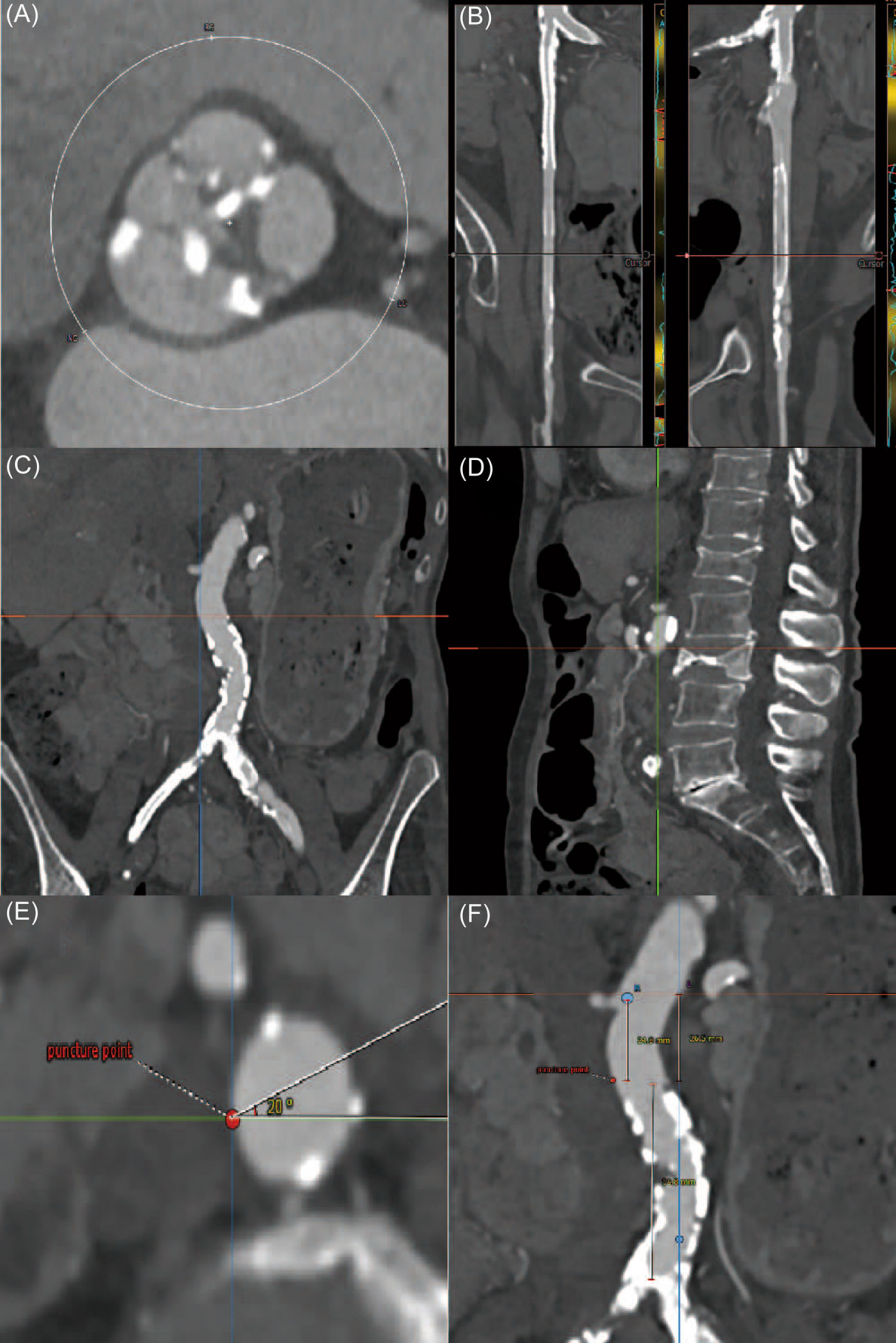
Pre‐TAVI CT evaluation. Pre‐TAVI CT showed Type‐1 bicuspid aortic valve (L−R) (A) and invalid transfemoral access (B). The ideal cava‐aorta puncture site was defined in the nearest location between IVC and aorta without calcification in the aortic wall (C). The upper part of L3 was defined as the bone marker for a puncture (D). The angle of the perpendicular puncture was measured to predict the long axis and short axis projections (E). The distances of the puncture site to renal arteries and iliac bifurcation were measured to ensure enough length for an emergent stent implant (F). CT, computed tomography; IVC, inferior vena cava; TAVI, transcatheter aortic valve implantation
